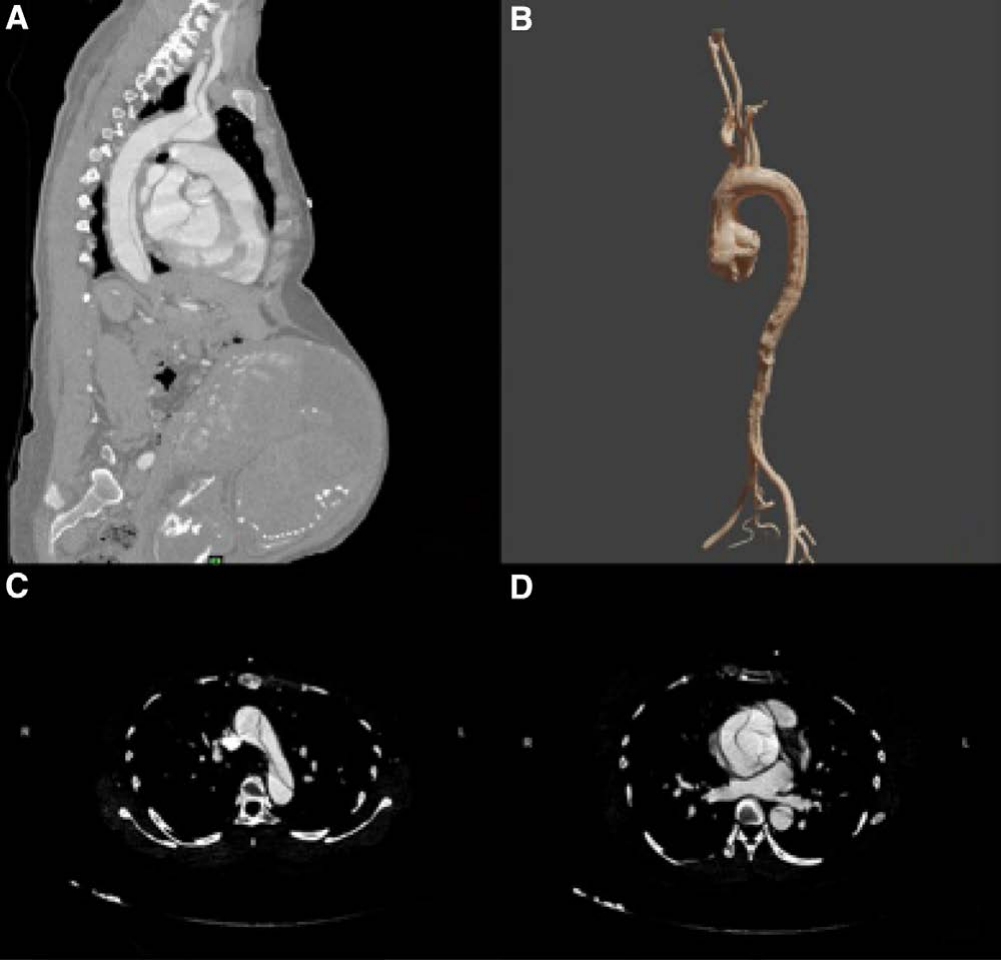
Preoperative CT showed a Standford A dissection, involving 3 branches of the aortic arch (A, B); The ascending aorta and aortic root were significantly widened the aortic valve is involved (C, D). CT = computed tomography.
